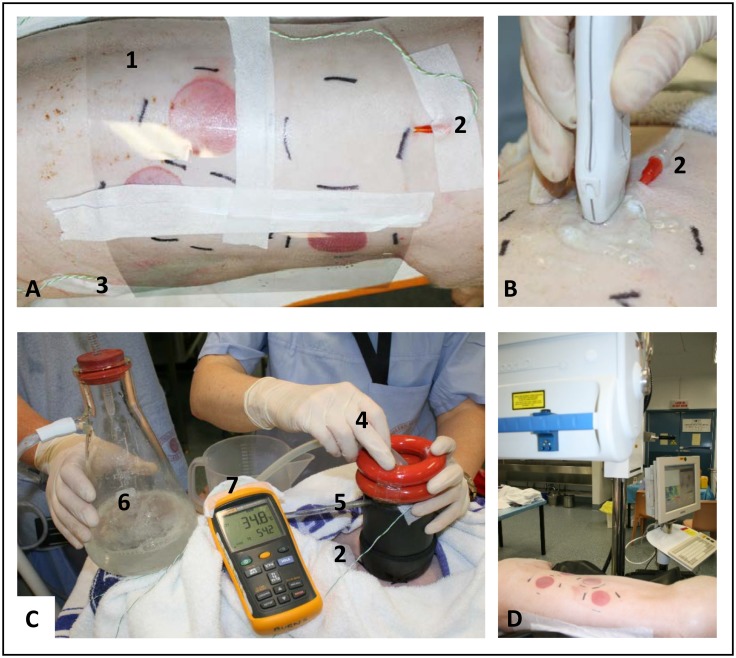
Scald burn creation and evaluation. A. preparation of the site for scalding, B. ultrasound assessment of subdermal temperature probe depth prior to scalding, C. scald burn creation and D. post-burn evaluation with Laser Doppler Imaging. 1. Shows protective plastic covering surrounding non-burn areas. 2. Subdermal temperature probe. 3. External skin temperature probe 4. Hot water inflow. 5. Suction water out. 6. Suction collection flask. 7. Fluke thermometer.
